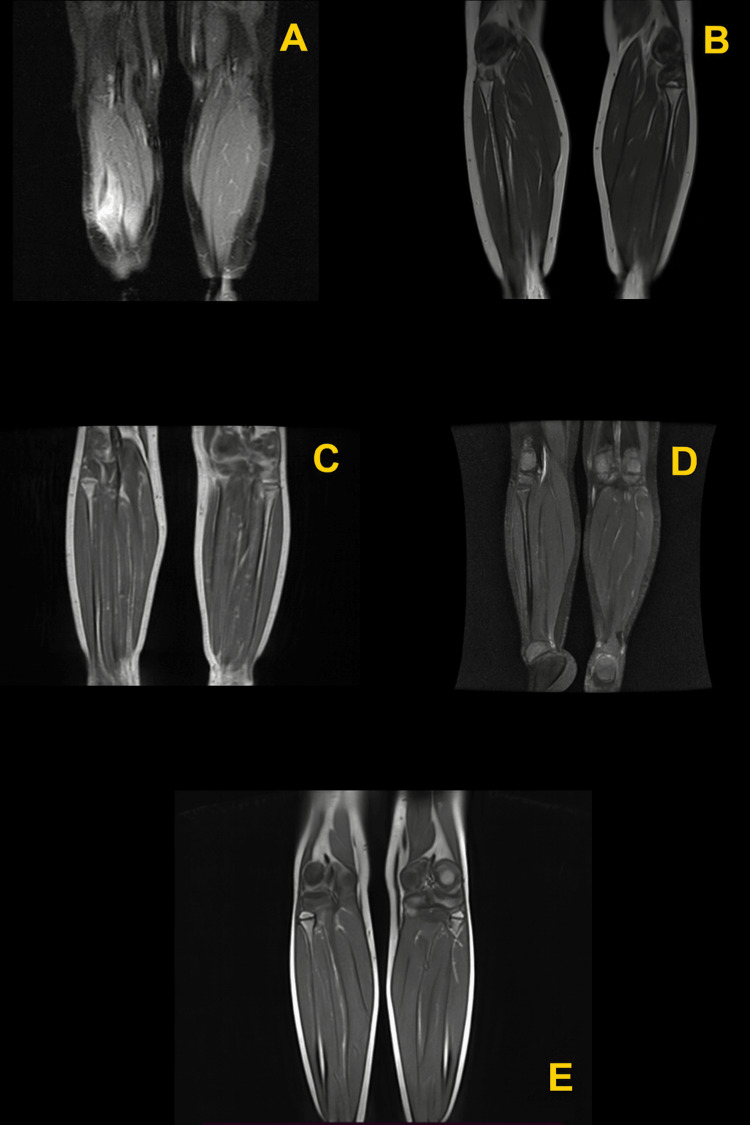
MRI with contrast of the lower extremities A. after initial chemotherapy; B. after continuation therapy part 1; C. after continuation therapy part 2; D. six months after end of therapy; E. 2.5 years after end of therapy
